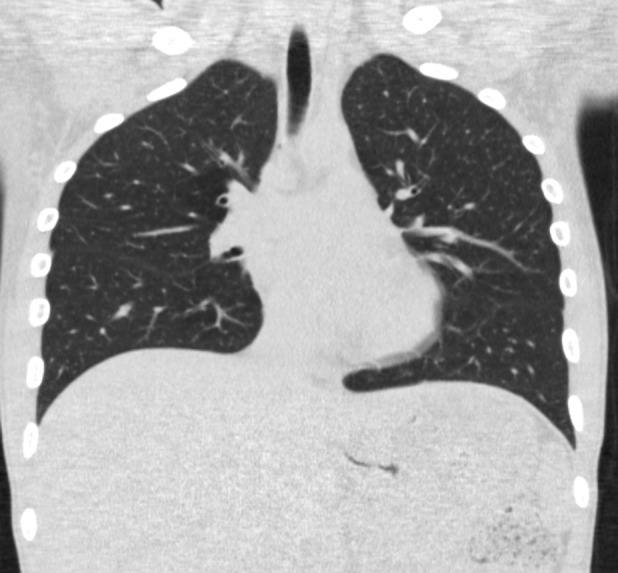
Coronal computed tomography scan, low dose (Toshiba Aquilion 64, acquisition parameters: 120 kV, 20 mA) shows full regression of the mediastinal emphysema.
